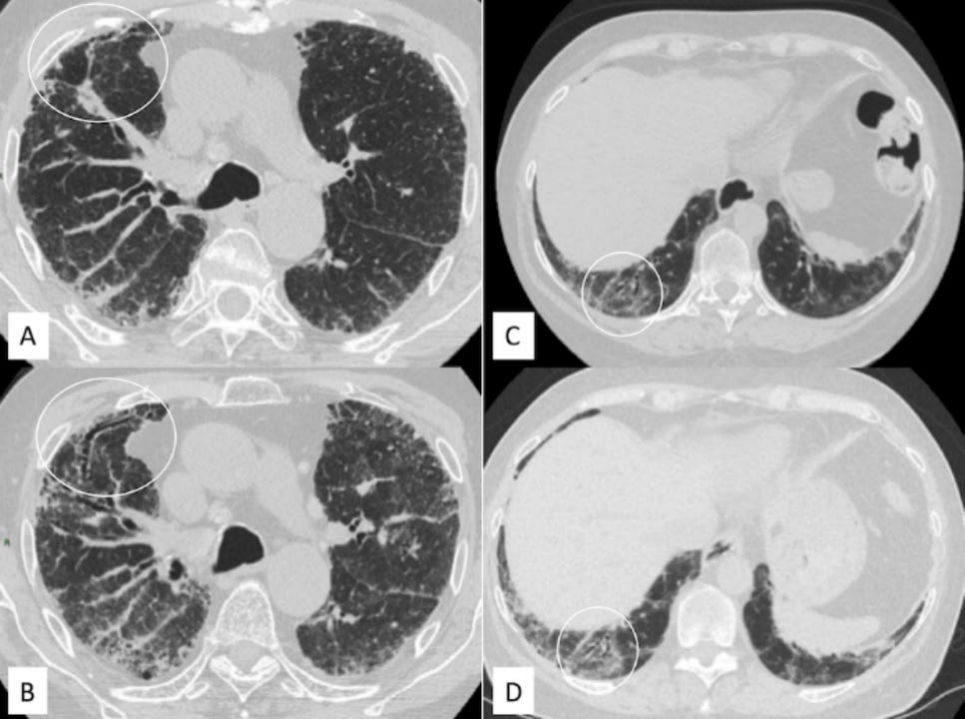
Traction bronchiectasis and stable bronchiectasis. A, B Traction bronchiectasis (white circles), which are increased in extension and caliper in the follow-up examination. C, D Stable bronchiectasis of the right lower lobe between high-resolution computed tomography examinations, even if progression is clearly demonstrated by increased representation of ground-glass opacification close to bronchiectasis
